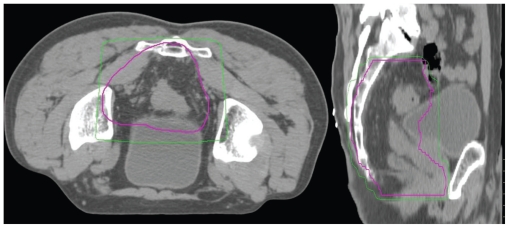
Axial and Sagittal phase II planning CT showing planning target volume (thick line) and 95% isodose (thin line). Note a 2 cm margin around the gross tumour volume (including nodal disease in the mesorectum), with anterior sacrum included.
